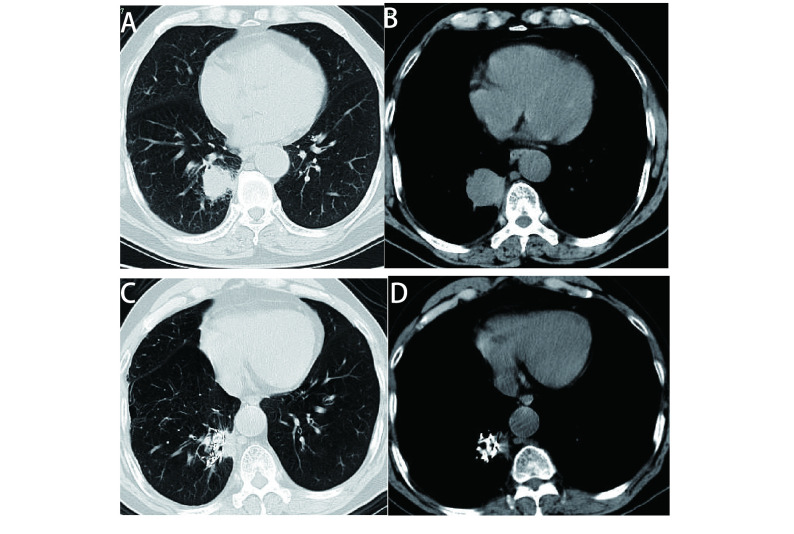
患者男，75岁，右下肺癌。A：CT肺窗示微波消融及粒子植入前的肿瘤病变；B：CT纵隔窗示微波消融及粒子植入前的肿瘤病变；C：微波消融术及粒子植入术后1个月，CT肺窗示肿瘤病灶较前缩小；D：微波消融术及粒子植入术后1个月，CT纵隔窗示肿瘤较前缩小，病灶内见空洞形成。 A 75 year man with right lower lung cancer. A: CT lung window showed tumor lesions before microwave ablation and particle implantation; B: CT mediastinal window showed tumor ablation before microwave ablation and particle implantation; C: One month after microwave ablation and particle implantation, the CT lung window showed a smaller tumor lesion than before; D: One month after microwave ablation and particle implantation, the CT mediastinal window showed that the tumor was smaller than before, and a cavity was formed in the lesion. CT: computed tomography.
